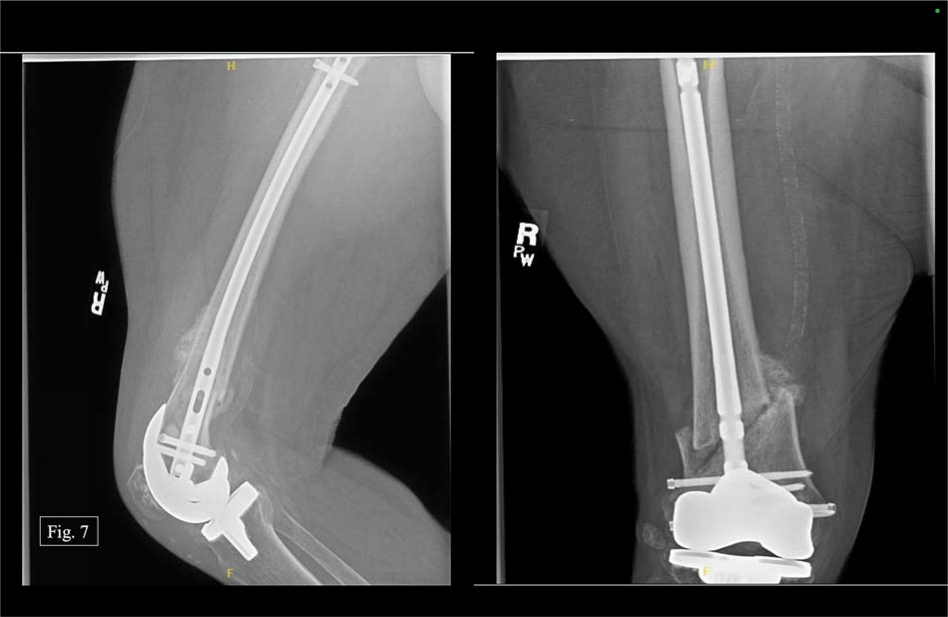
Right, 3 month postoperative AP and lateral X-rays.
